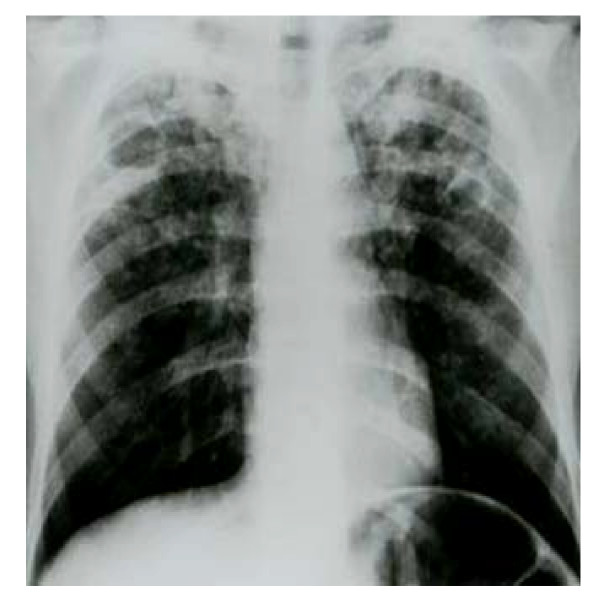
Anteroposterior chest radiograph showing cavitary lesions in both lungs
